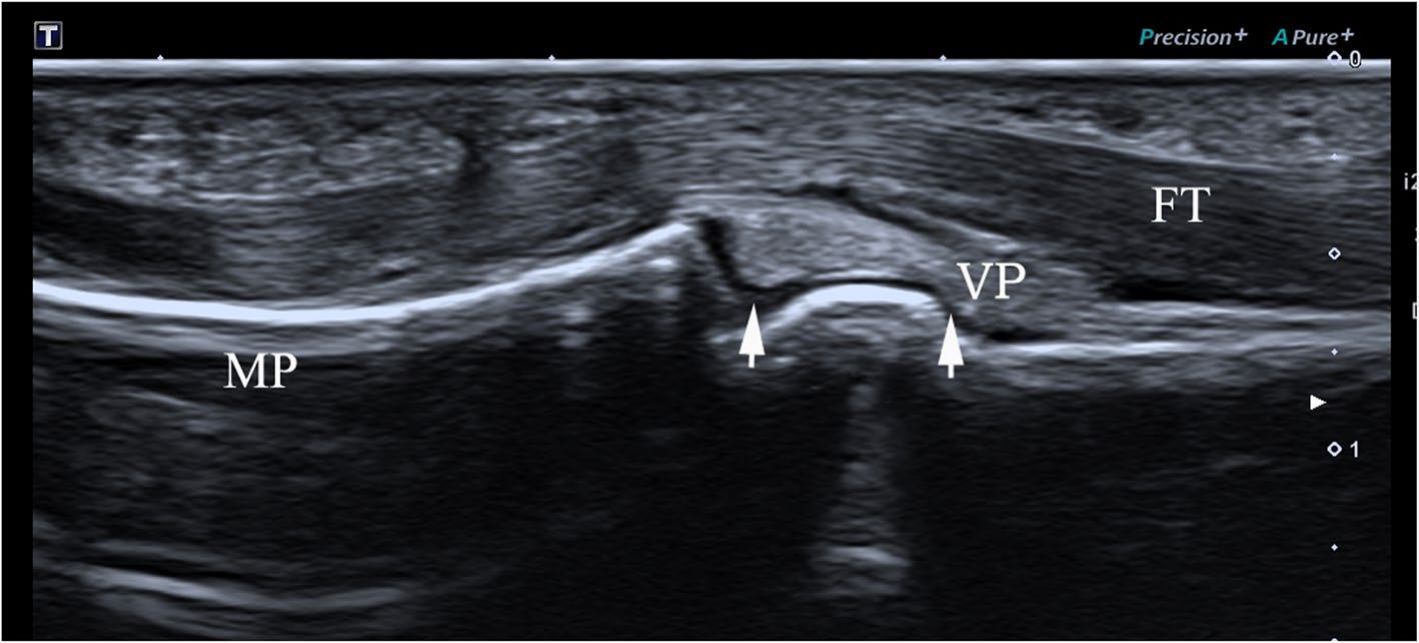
High frequency ultrasonography image of normal volar plate at PIP of a 30-year-old male in longitudinal plane(arrow). VP: volar plate; FT: flexor tendon; MP: middle phalanx
